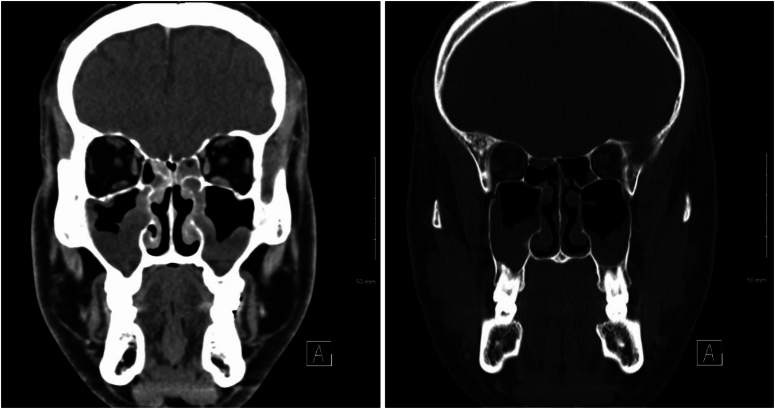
CT maxillofacial with IV contrast: opacification of the frontal and ethmoid sinuses suggestive of sinusitis. Paranasal sinus polypoid soft tissue densities indicate possible sinonasal polyposis or retention cysts.
